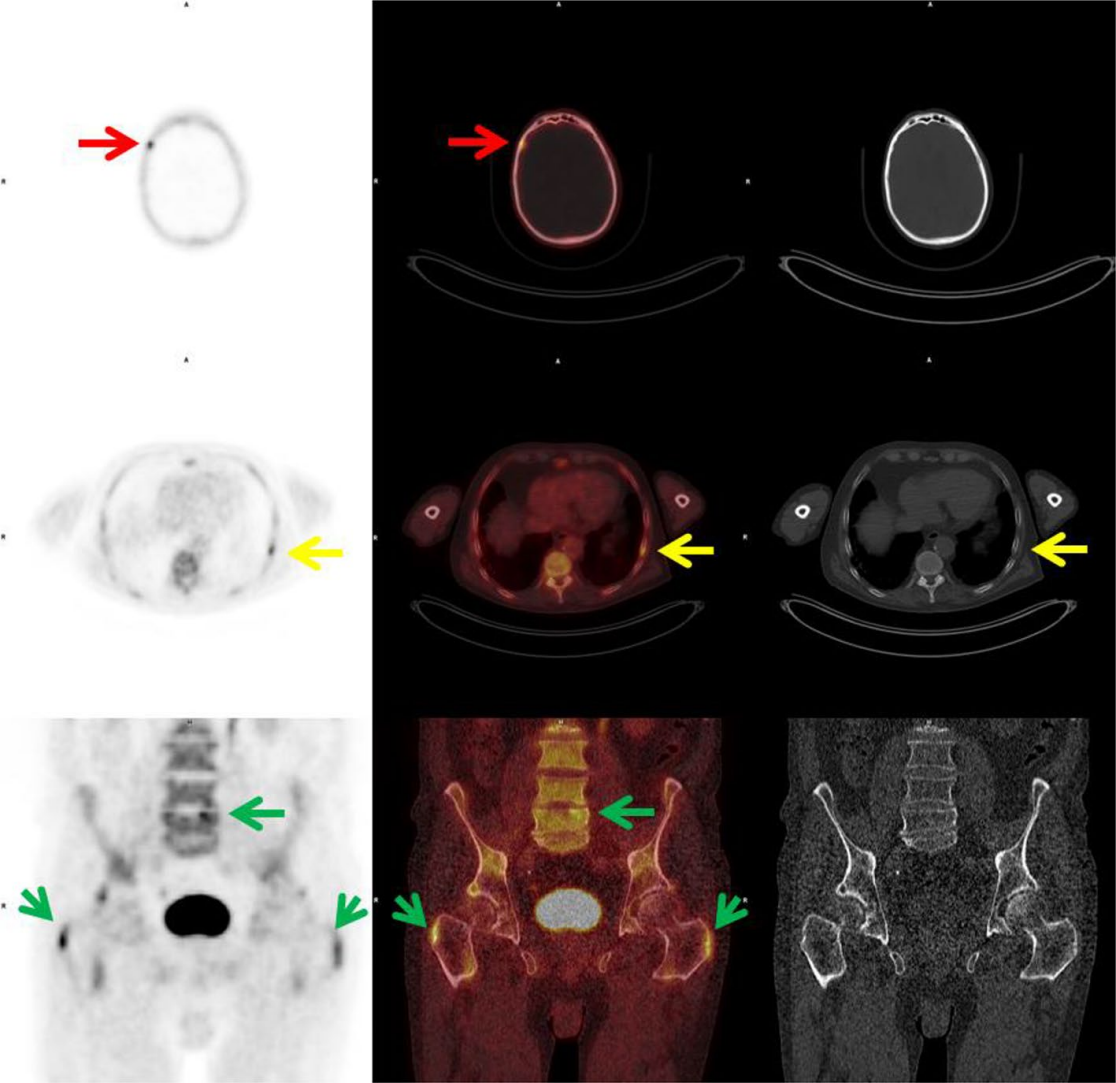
^18^F-NaF PET, PET/CT and CT images showing increased ^18^F- uptake in the right side of the os frontalis without substrate on CT, unlikely for bone metastasis (red arrows). Increased ^18^F- uptake in the right 8th rib, with faint sclerosis on CT, equivocal for bone metastasis (yellow arrows). Increased ^18^F- uptake in degenerative changes in the lumbar spine and enthesopathy in the trochanteric region at both sides (green arrows). 83-years old patient abstained from therapy with curative intent and androgen deprivation therapy was initiated
